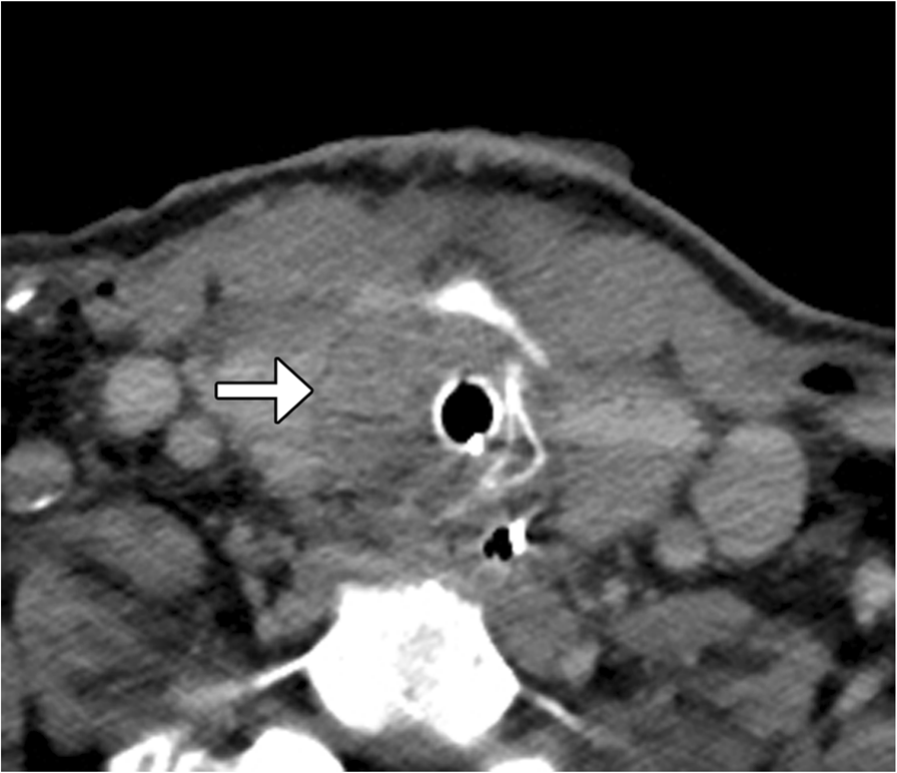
Laryngeal neuroendocrine carcinoma. Axial CT image shows an infiltrative right laryngeal mass with extralaryngeal extension (arrow)
